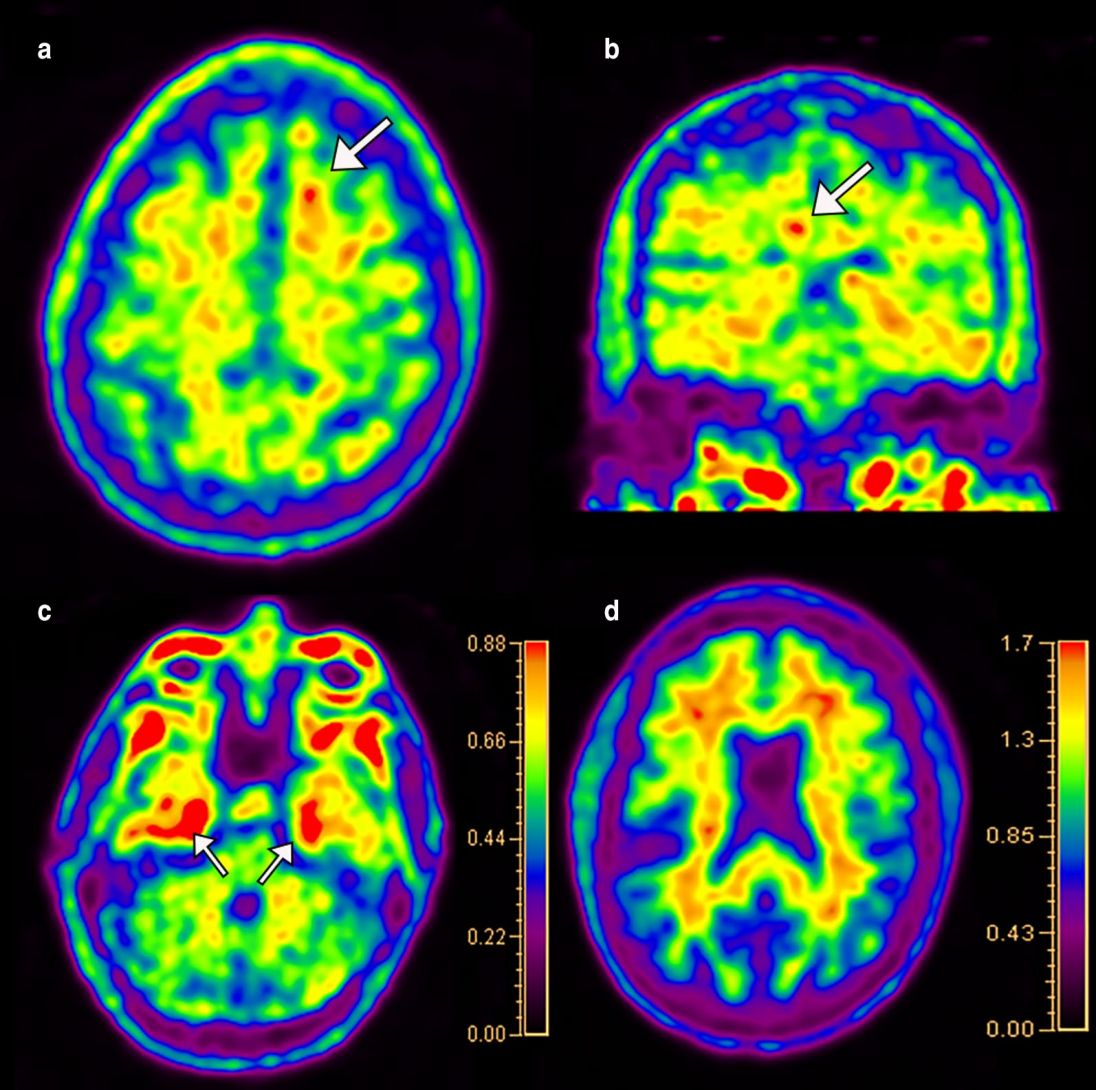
A 70-year-old man with history of military combat and traumatic brain injury with MCI, MoCA 26/30. Flortaucipir PET/CT demonstrates regions of tau retention in the sulcal depths of the parasagittal frontal cortices (arrows in a and b). Tau retention is also noted in the bilateral mesial temporal lobes (arrows in c). Florbetapir PET/CT (d) demonstrates no significant cortical amyloid uptake involving the parietal lobes. Given the findings on PET/CT memory impairment was diagnosed as related to CTE
